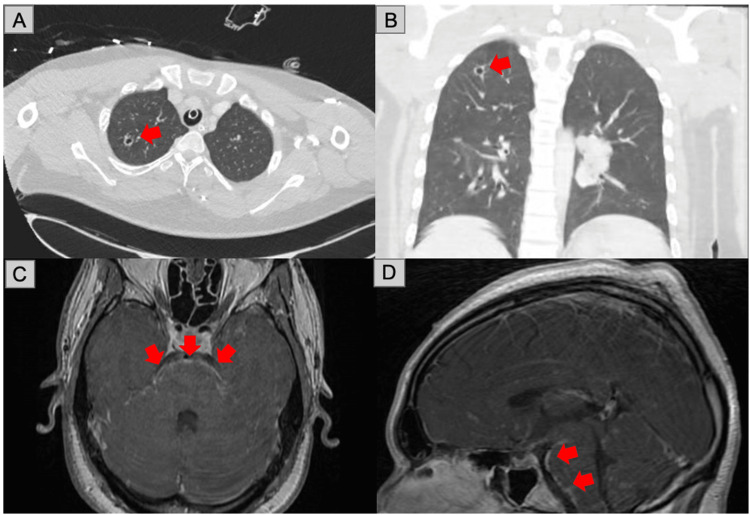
Radiologic evaluation (A) Axial CT of the chest with contrast (arrow). (B) Coronal CT of the chest with contrast: mediastinal and bilateral hilar lymphadenopathy; cavitary and spiculated nodules in the right upper and middle lobes, the largest measuring 1.2 cm in the right upper lobe (arrow). (C) Axial T1-weighted MRI with contrast (arrows). (D) Sagittal T1-weighted MRI with contrast: abnormal leptomeningeal enhancement along the surface of the brainstem (arrows). CT: computed tomography, MRI: magnetic resonance imaging
